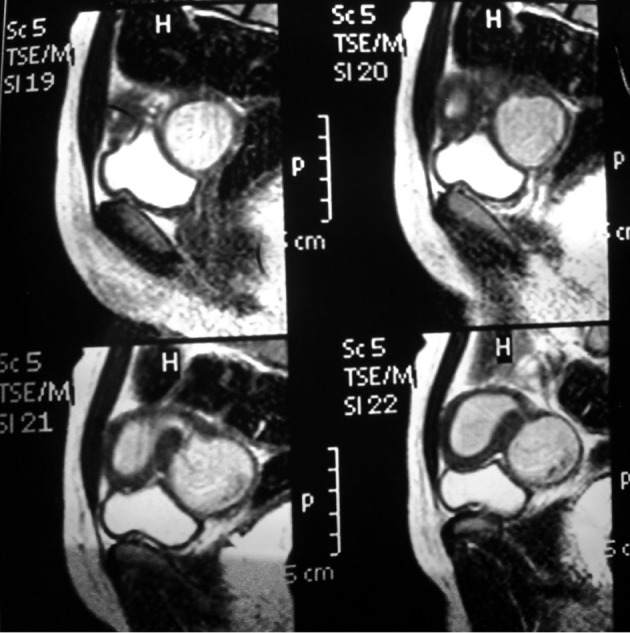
MRI images showing the hematometra along with the hematocolpos
